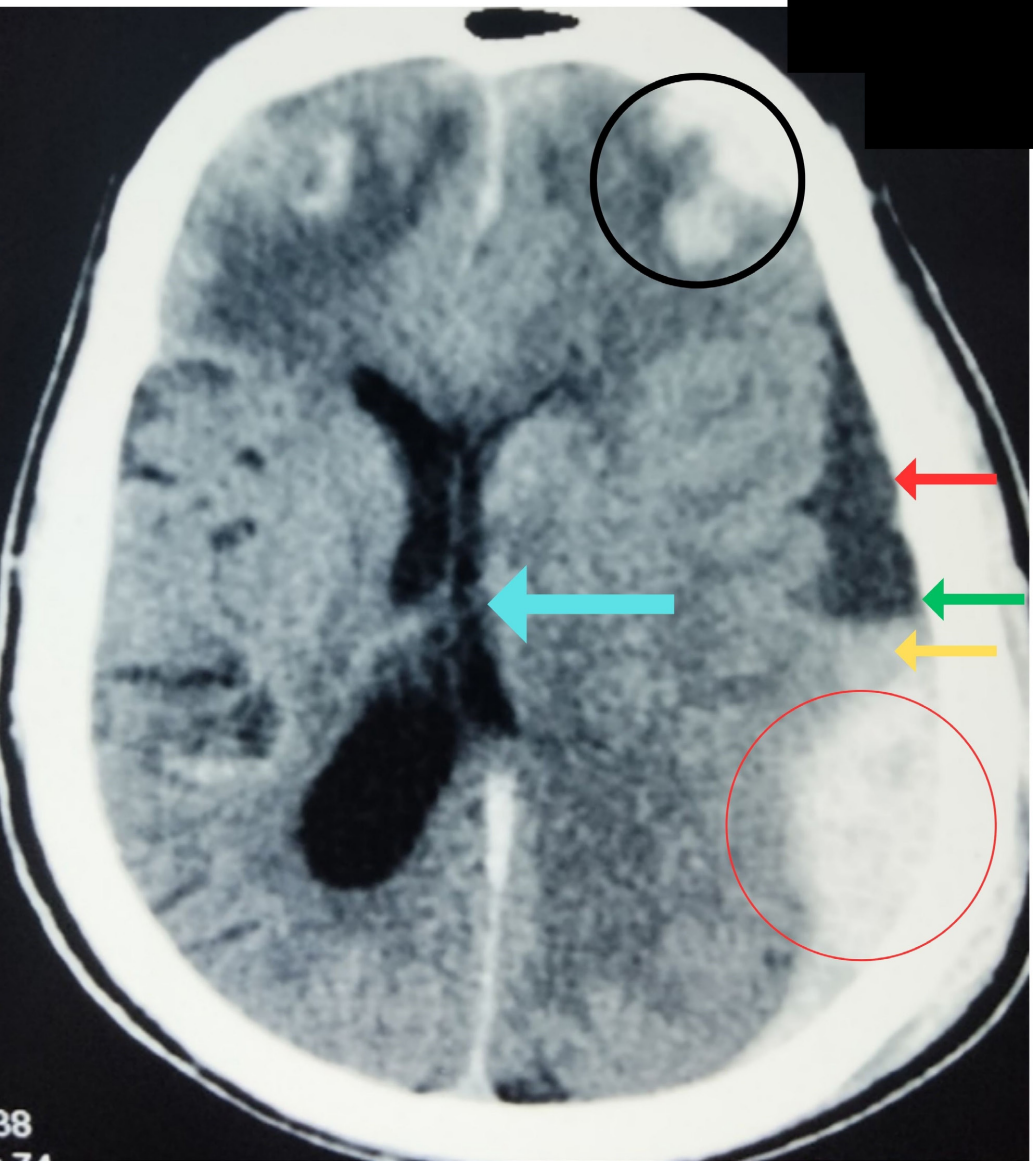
A computed tomography scan of the head showing contusions (black circle), a hypodense area (red arrow) with a fluid–fluid level (green arrow) and a hyperdense area below (yellow arrow) it with rightward midline shift (blue arrow) suggesting acute‐on‐chronic subdural hematoma, and a hyperdense area suggesting extradural hematoma (red circle).
